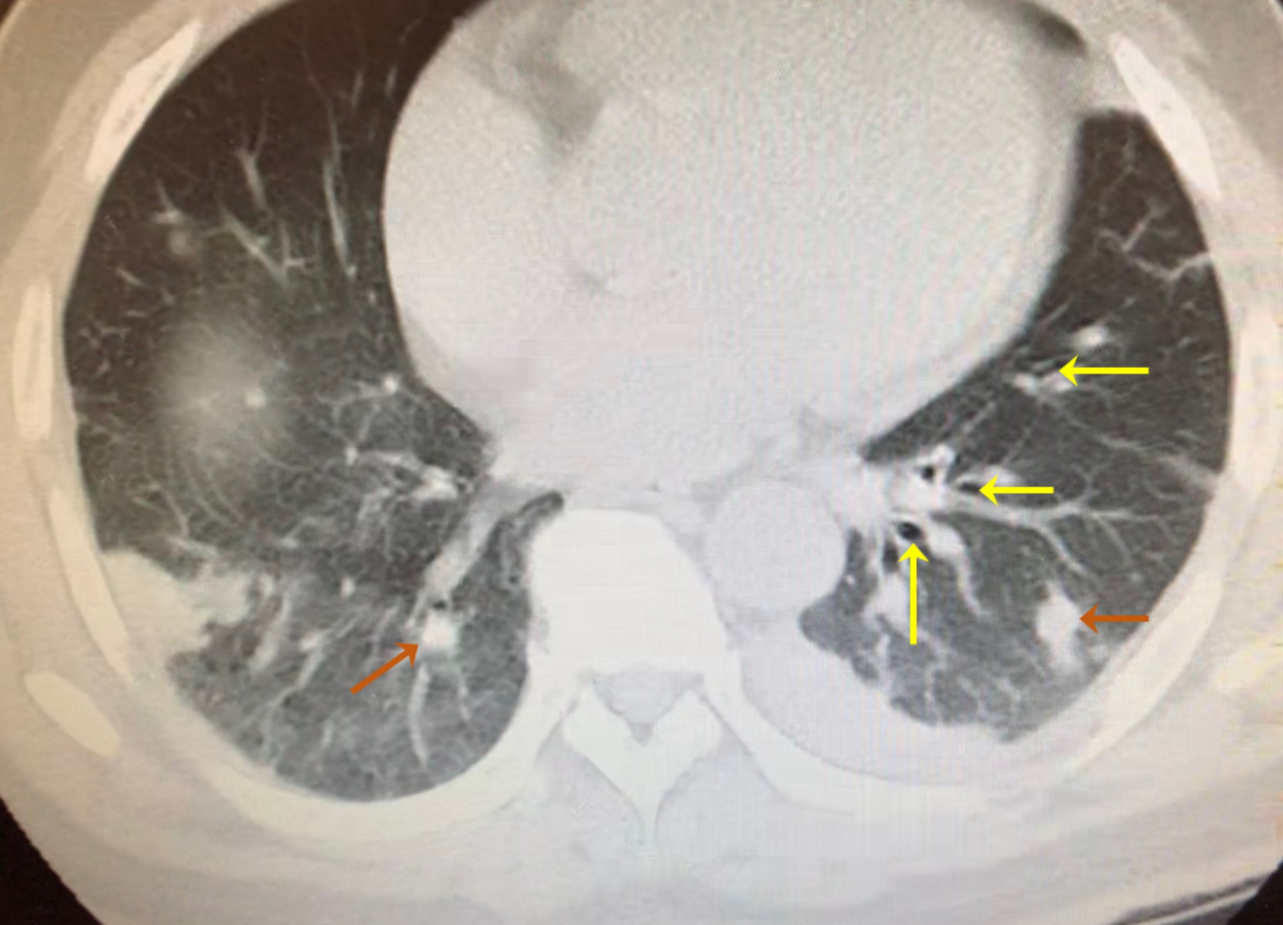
Chest CT of the patient at the time of tuberculosis diagnosis with air bronchograms (yellow arrows) and pulmonary nodules (orange arrows).
